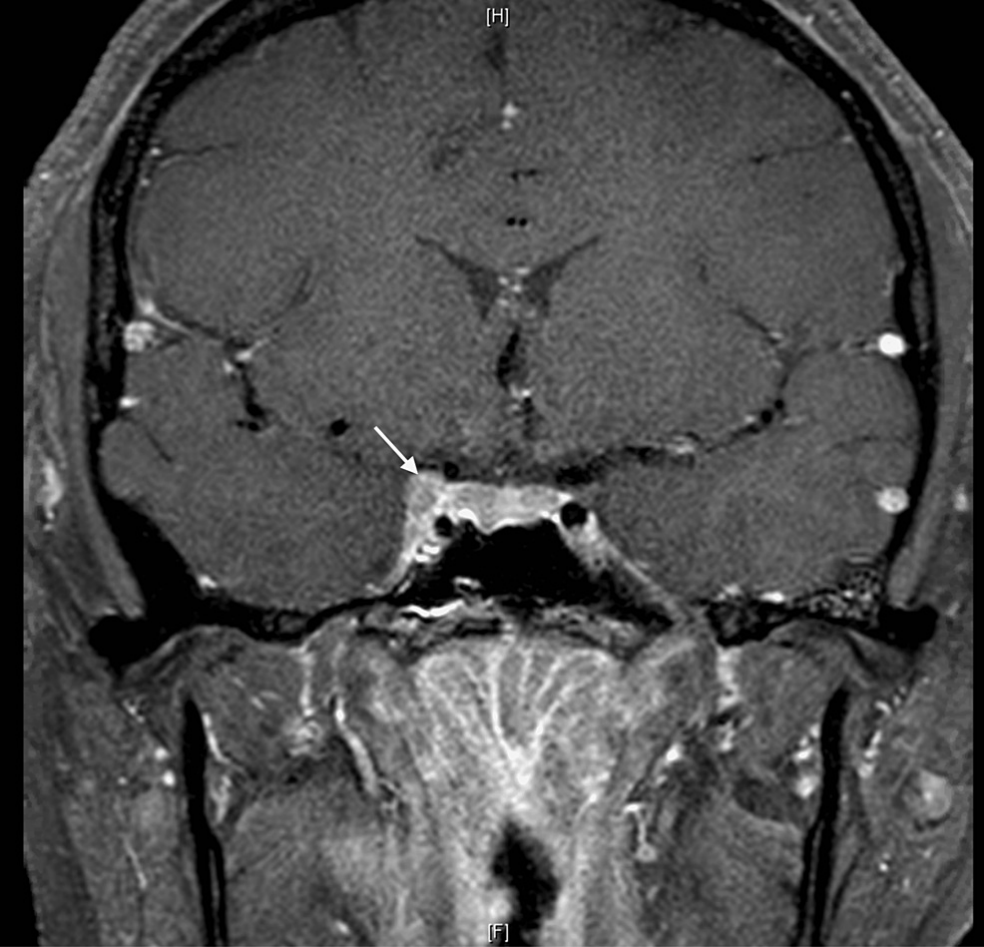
MRI of CSVT: The post-GAD T1 shows a possible filling defect within the right cavernous sinus (white arrow). MRI: magnetic resonance imaging CVST: cerebral venous sinus thrombosis GAD: Gadolinium contrast
